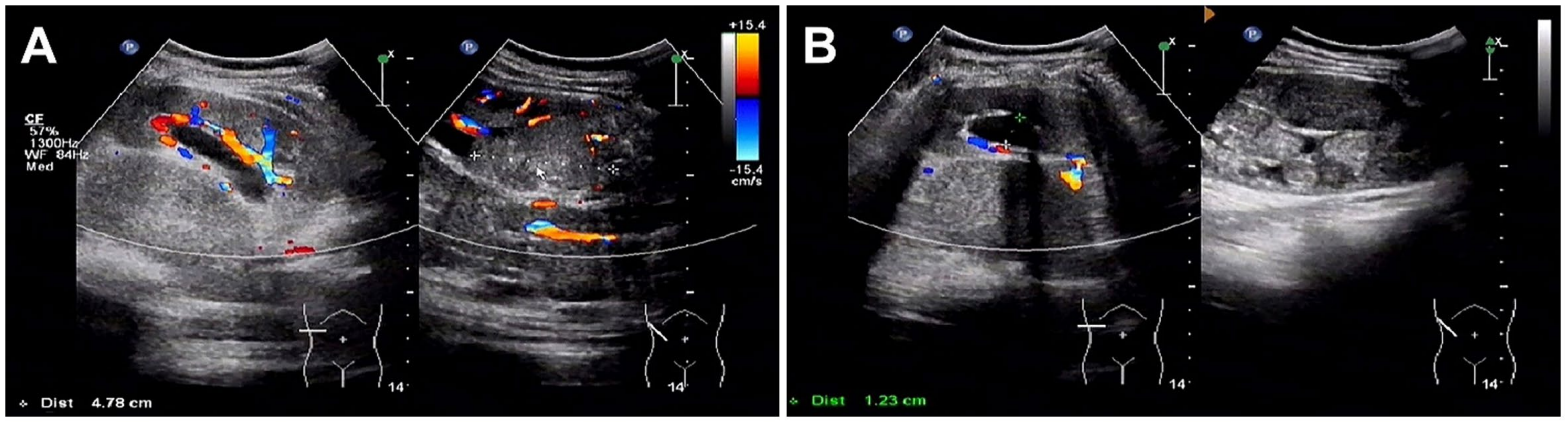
Ultrasound images of Xp11.2 translocation/TFE3 gene fusion associated with renal cell carcinoma (Xp11.2 RCC). (A) A highly heterogeneous hypoechoic mass in the right subrenal region with an irregular, lobulated morphology. The mass encroached on the renal sinus and protruded outward from the kidney. A class I blood flow signal was observed within and around the mass. (B) Multiple enlarged lymph nodes were detected adjacent to the right renal hilum, in proximity to the inferior vena cava, in the right adrenal region, and near the abdominal aorta.
